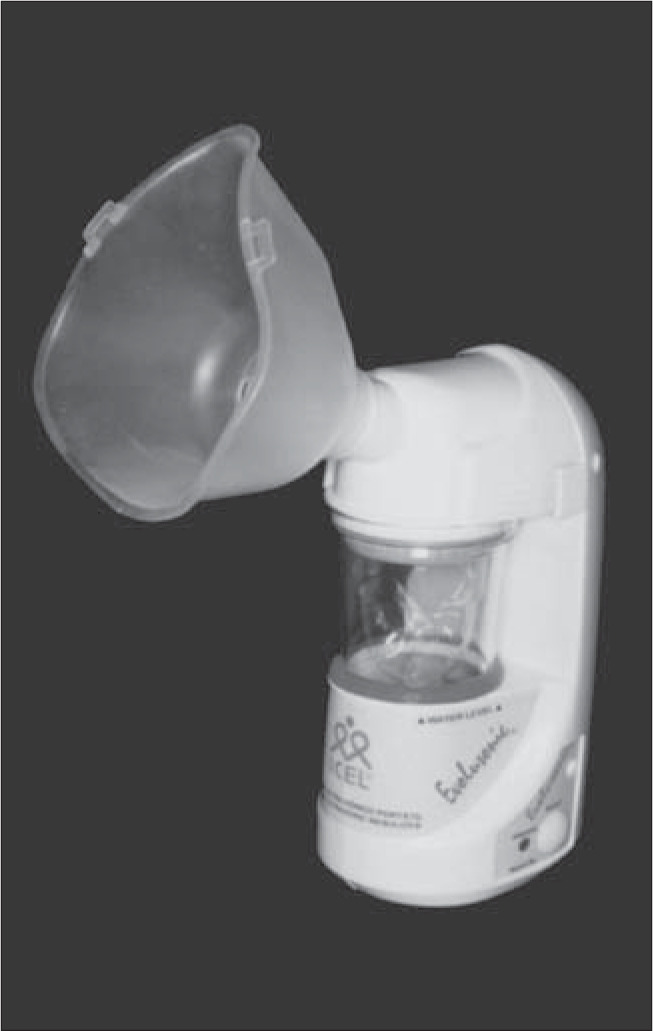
Icel ultrasonic nebulizer (model Evolusonic 1000BR).
